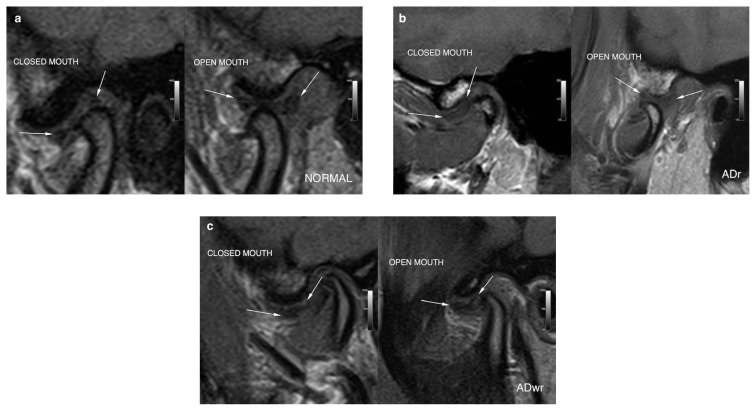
Classification of temporomandibular disc locations as normal (a), ADr (b), and ADwr (c) on sagittal oblique T1-weighted MR images.
